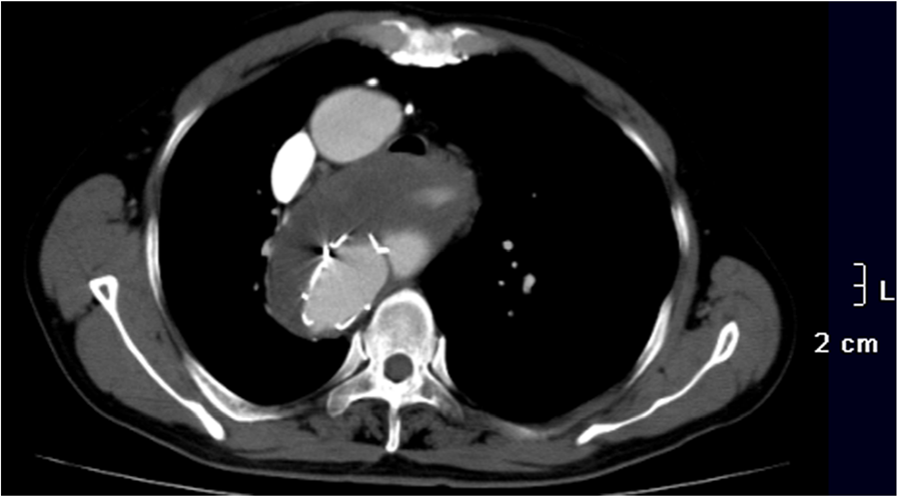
After 18 months follow-up, CTA documented that the false lumen was largely thrombosed only with a mild type II endoleak and a mild descending aortic expansion.
